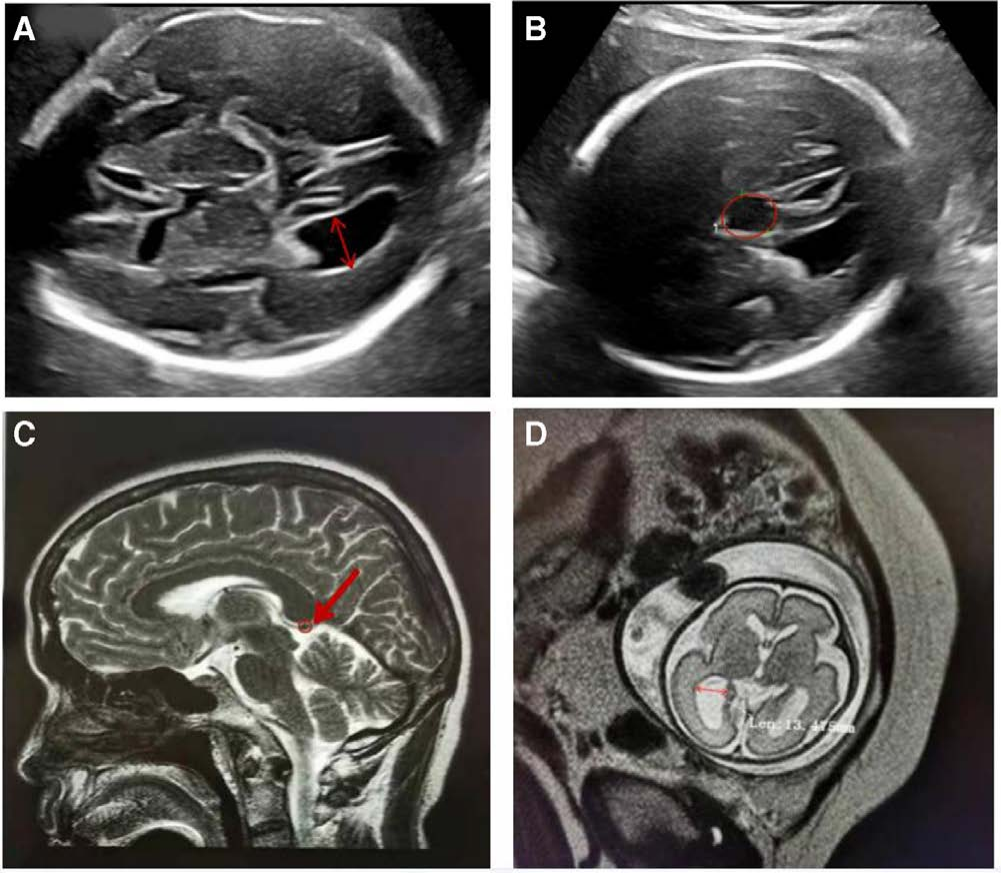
The fetal imaging results at 29 + 1 weeks of GA: (a) ultrasound showed the widening posterior horn of the left lateral ventricle (red arrow); (b) ultrasound showed intracranial arachnoid cystic structure (red circle); (c) midsagittal T2-weighted MRI image showed intracranial cyst arose from the enlarged cisterna venue magnate cerebri; (d) transverseT2-weighted MRI showed mild expansion of left ventricle.
